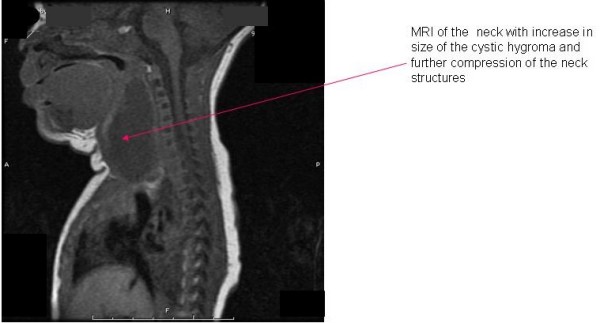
Follow-up magnetic resonance imaging of neck showing increase in size of the cystic hygroma with further compression of the structures of the neck.
